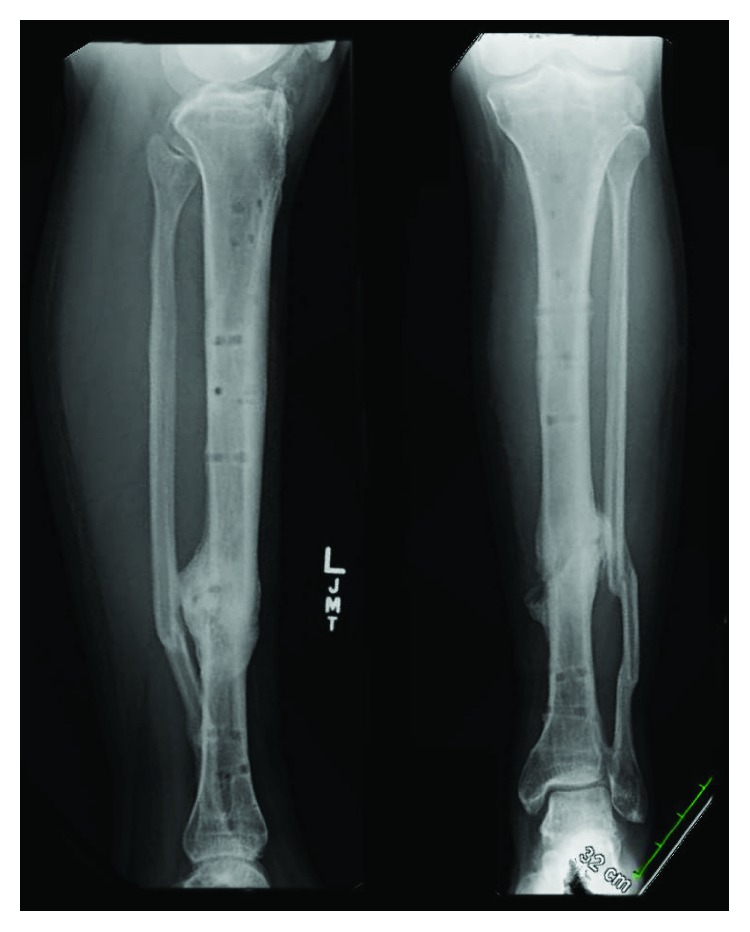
AP and lateral radiographs of the left leg show a united fracture at week 24.
